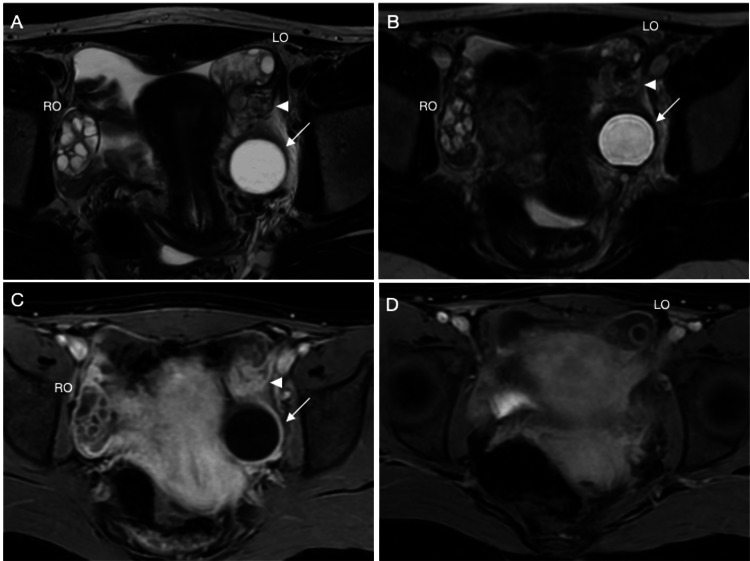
MRI T2 and post-contrast T1 images of isolated fallopian tube torsion T2 Images A and B in two consecutive levels show a normal right ovary with polycystic morphology, and the left ovary is pushed anteriorly with a parenchymal high T2 signal denoting edema. In the left adnexa posteriorly, there is a simple cyst (arrow); between the ovary and the cyst, there is a tortuous structure with an intermediate T2 signal (arrowhead), which turned out to be a twisted fallopian tube. Post-contrast T1 Images C and D in two consecutive levels show again the normally enhancing right ovary in Image C, and the left ovary shows follicular rim enhancement and peripheral enhancement of the left ovary as shown in Image D, which, along with edema, raised suspicion for a twisted left ovary. The twisted fallopian tube showed good enhancement, as shown in Image C.
